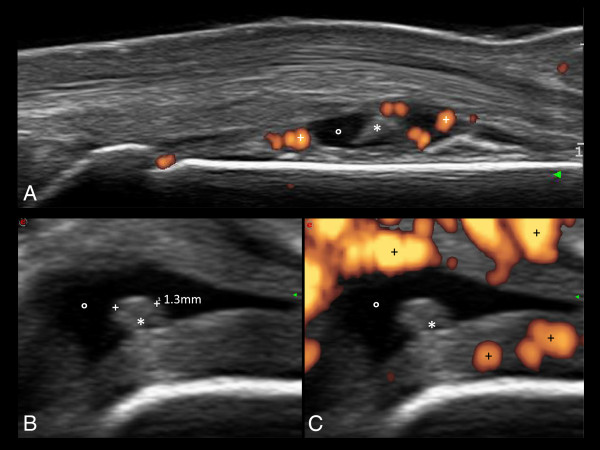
Early arthritis. The longitudinal dorsal scan of the II metacarpophalangeal joint (A) shows a wide spectrum of inflammatory findings, such as joint cavity widening, fluid collection (°), synovial hypertrophy (*) and multiple power Doppler spots (+). The transverse scans of the same joint (B, C) better confirm the presence of a highly perfused synovial pannus that is a strong predictor of anatomical damage. This figure is original and has not been previously published.
